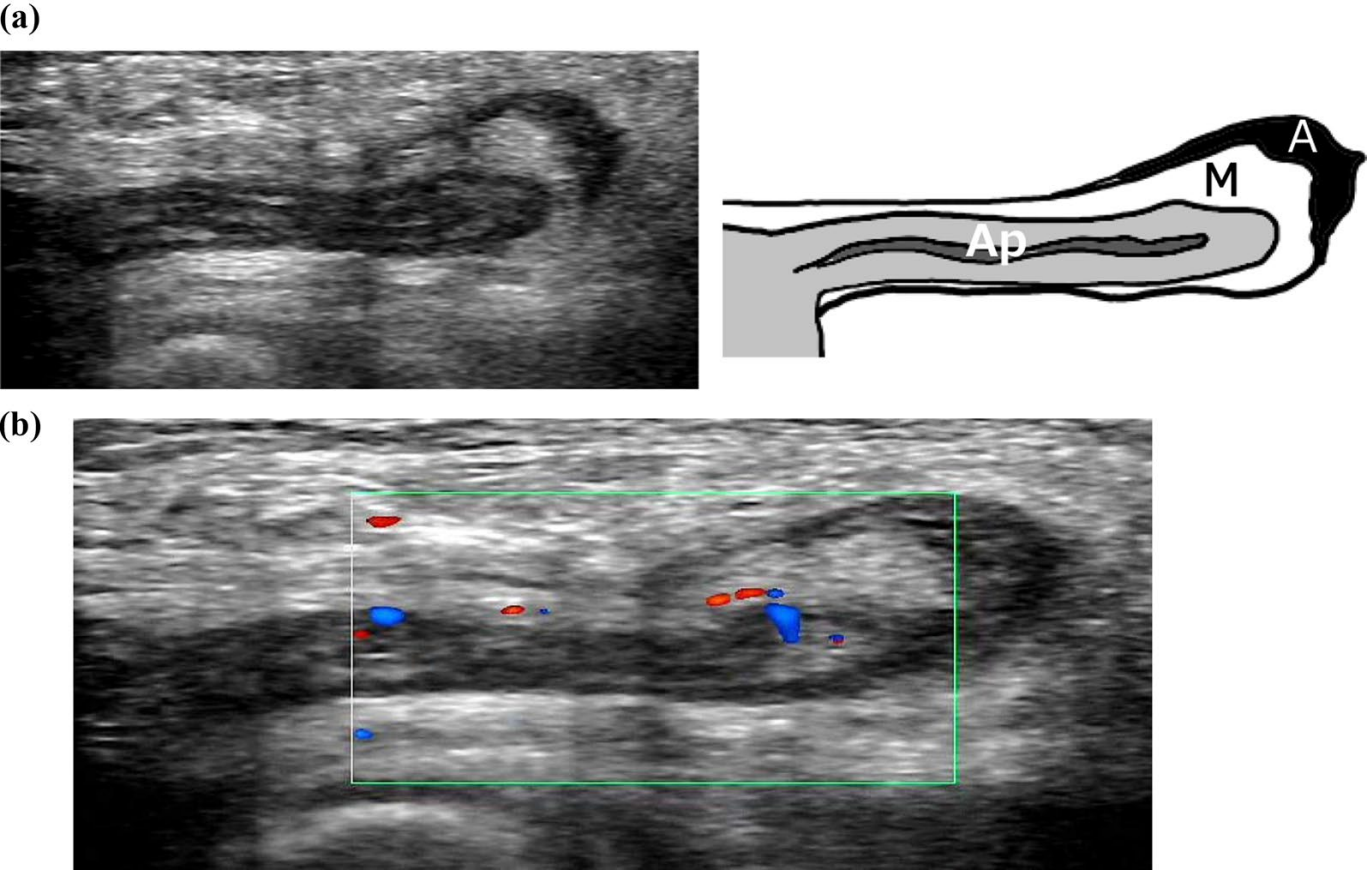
Ultrasonography (US) (Case 2). a B-mode US showing a blind-ended isoechoic tubular structure (5 mm in diameter) continuous with the cecum, a surrounding reticular hyperechoic area, and an anechoic area on the medial side of the right femoral vein. They were diagnosed as appendix (Ap), mesoappendix (M), and ascites (A), respectively. b Color doppler US showing pulsatile blood flow signals in the appendiceal wall
